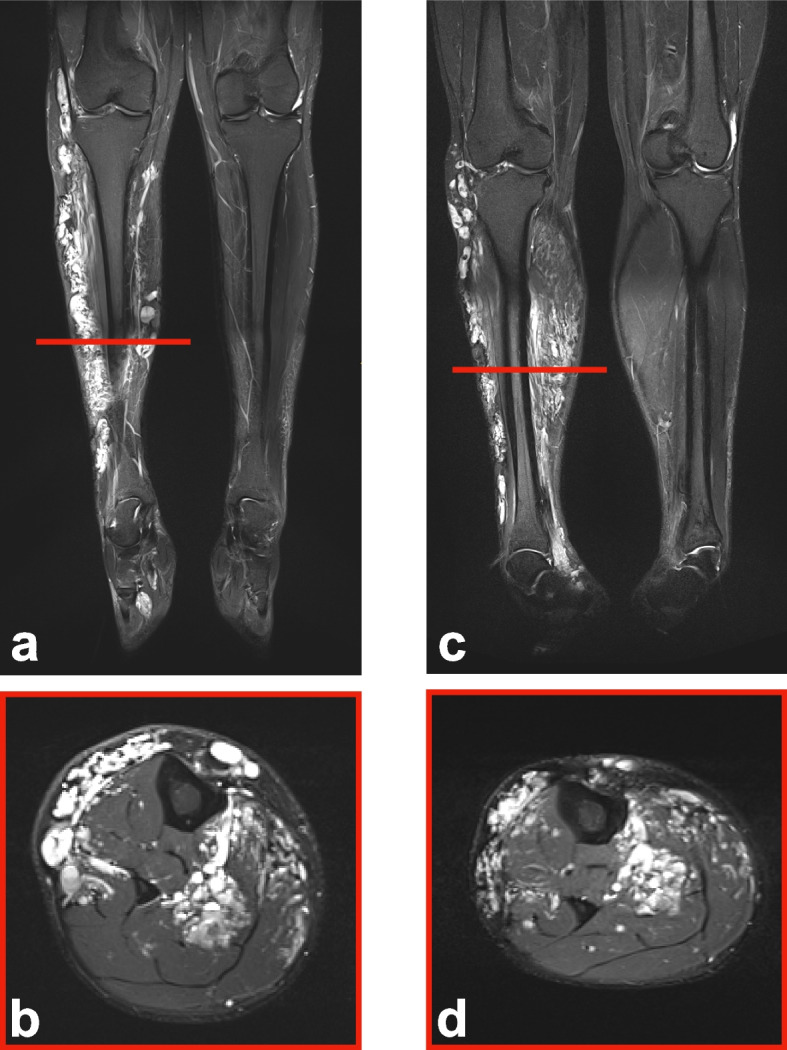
MRI imaging of venous malformation before and after BEST therapy. MRI depicting the case of a 27-year-old patient with a venous malformation on his right lower leg, treated with BEST. a Coronal MRI scan before treatment showing the lesion as a hyperintense area. b Axial MRI scan depicting the venous malformation at its maximum extent. c Coronal MRI scan of the leg obtained 4 months post-therapy, showing a reduction in lesion volume after just one therapy session, with notable decrease of the hyperintense area. d Axial MRI scan of the leg
